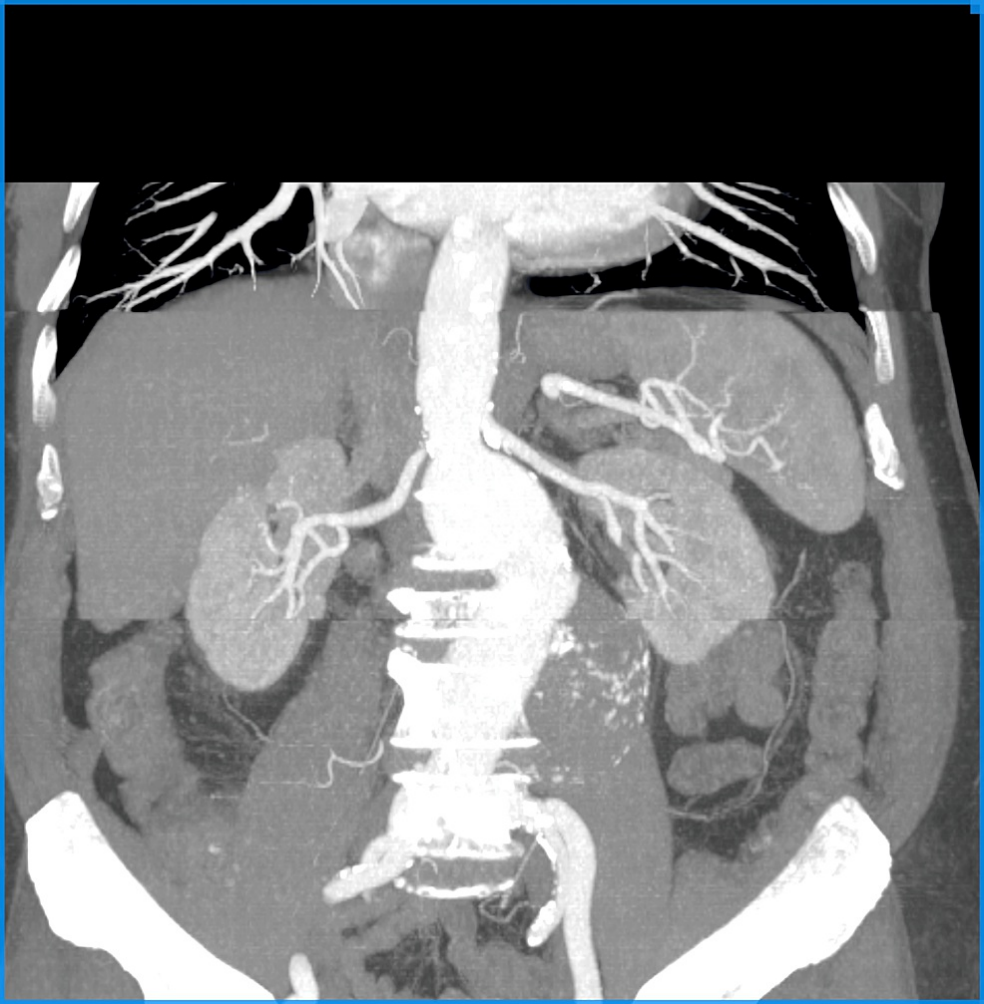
Case 1: Preoperative CTA. CTA, computed tomography angiography
